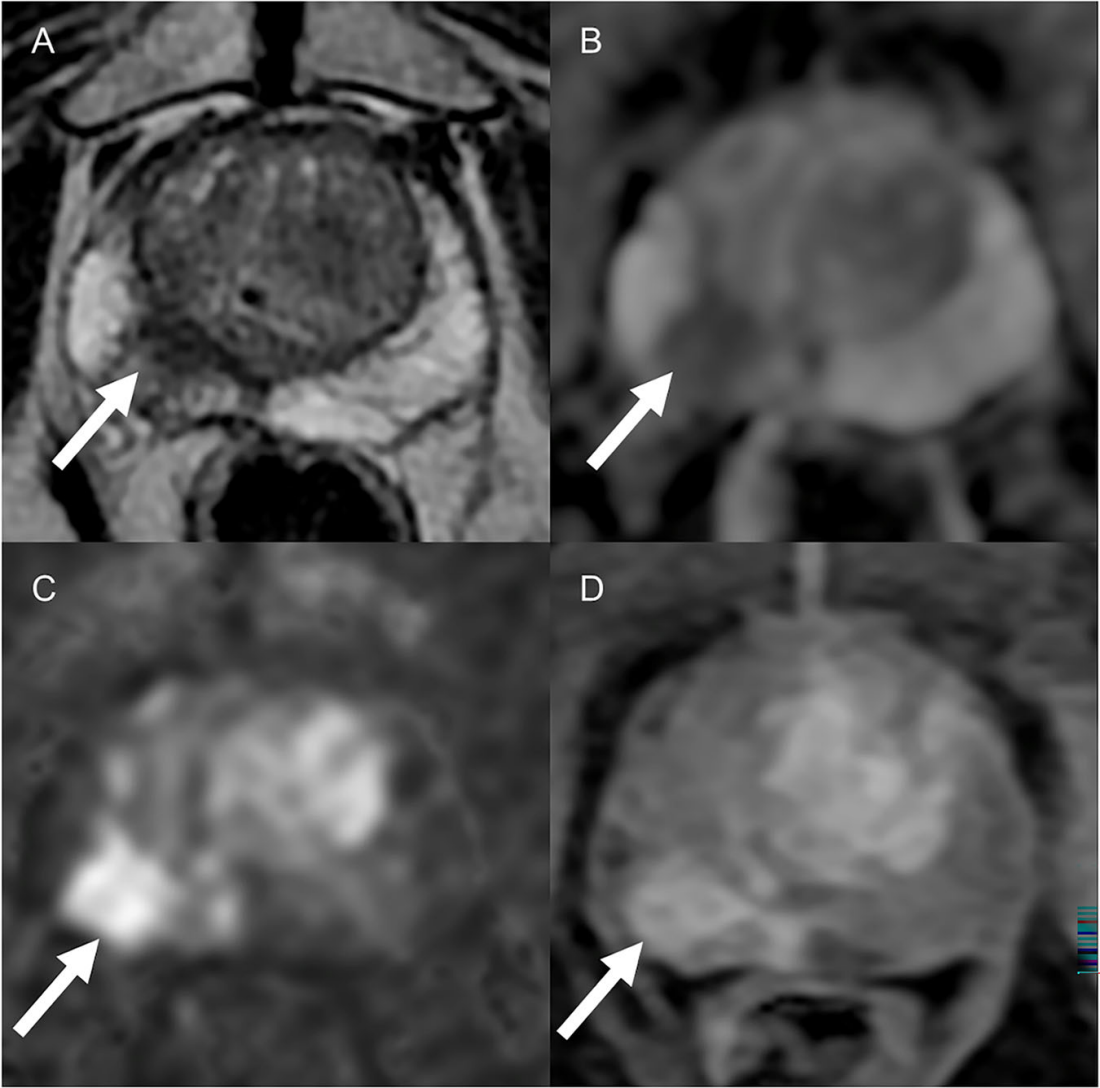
The T2-weighted axial image detects a lesion in the peripheral zone of the right lobe (A). The ADC (B) and DWI (C) maps demonstrate a focal area of markedly hypointense/hyperintense signal in the ADC map and high b-value, with corresponding positive DCE findings (D), consistent with PI-RADS 5 (arrows)
